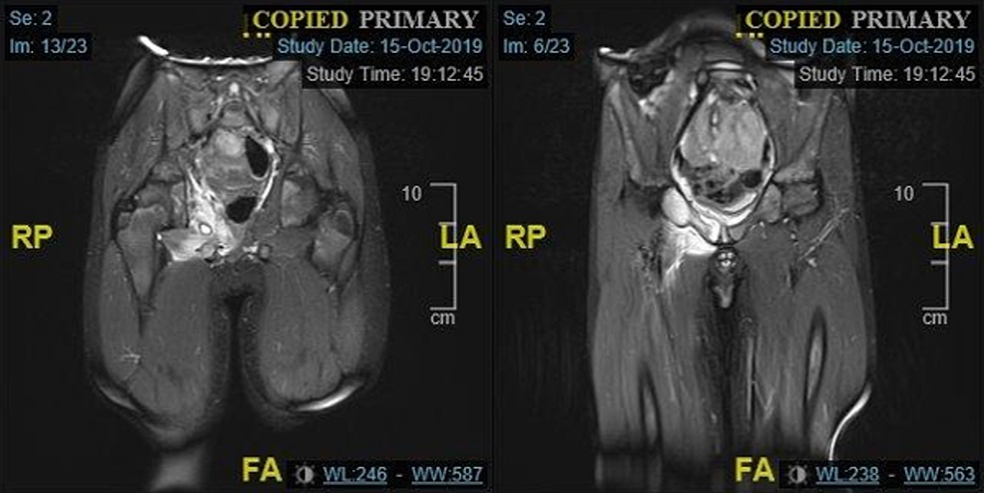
Coronal sections from T2-weighted MRI showing extensive myositis in the right obturator internus containing a collection, less severe myositis in the obturator externus and pectineus muscle (left) and suspicion of osteomyelitis of right pubic bone (right).
